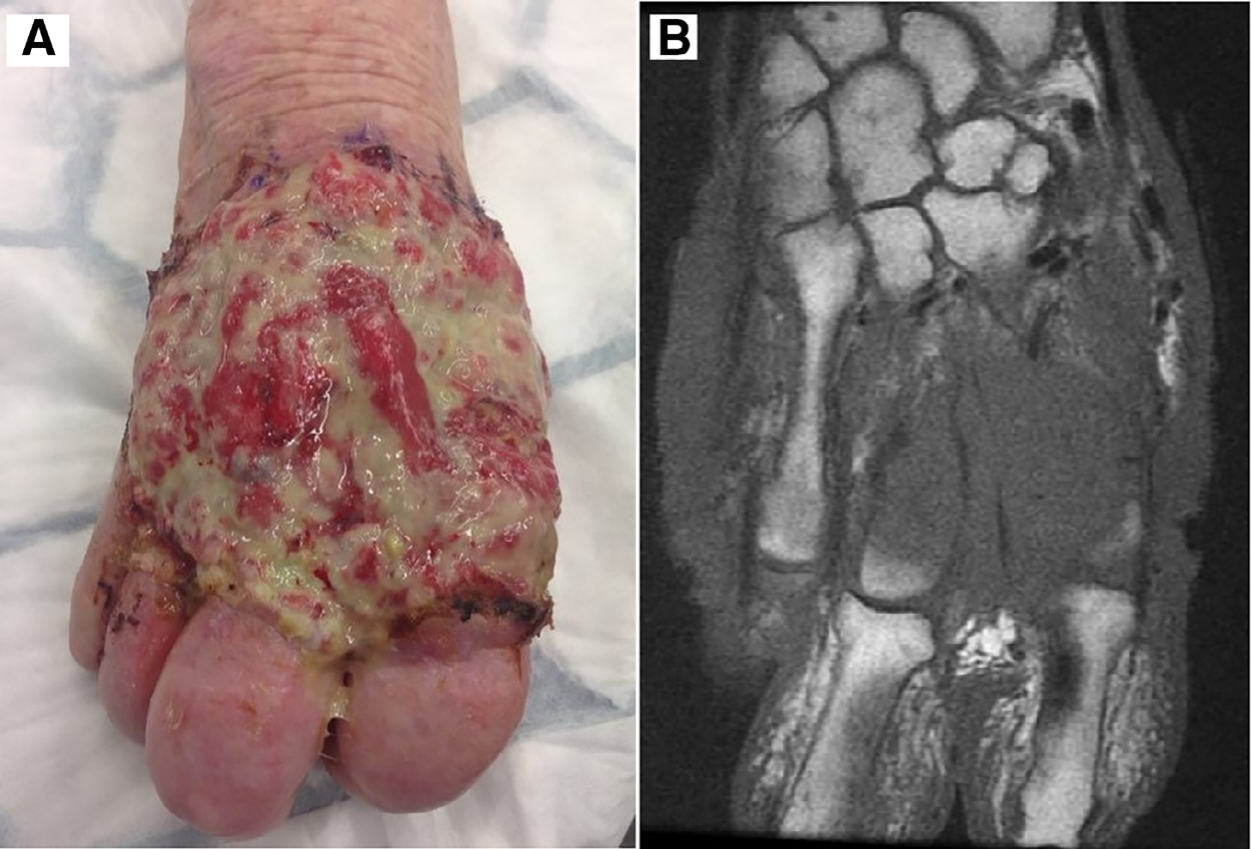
(A) Showing SCC involving dorsum of right hand for Case 1. (B) MRI showing tumour extension into index and middle metacarpal bones.
